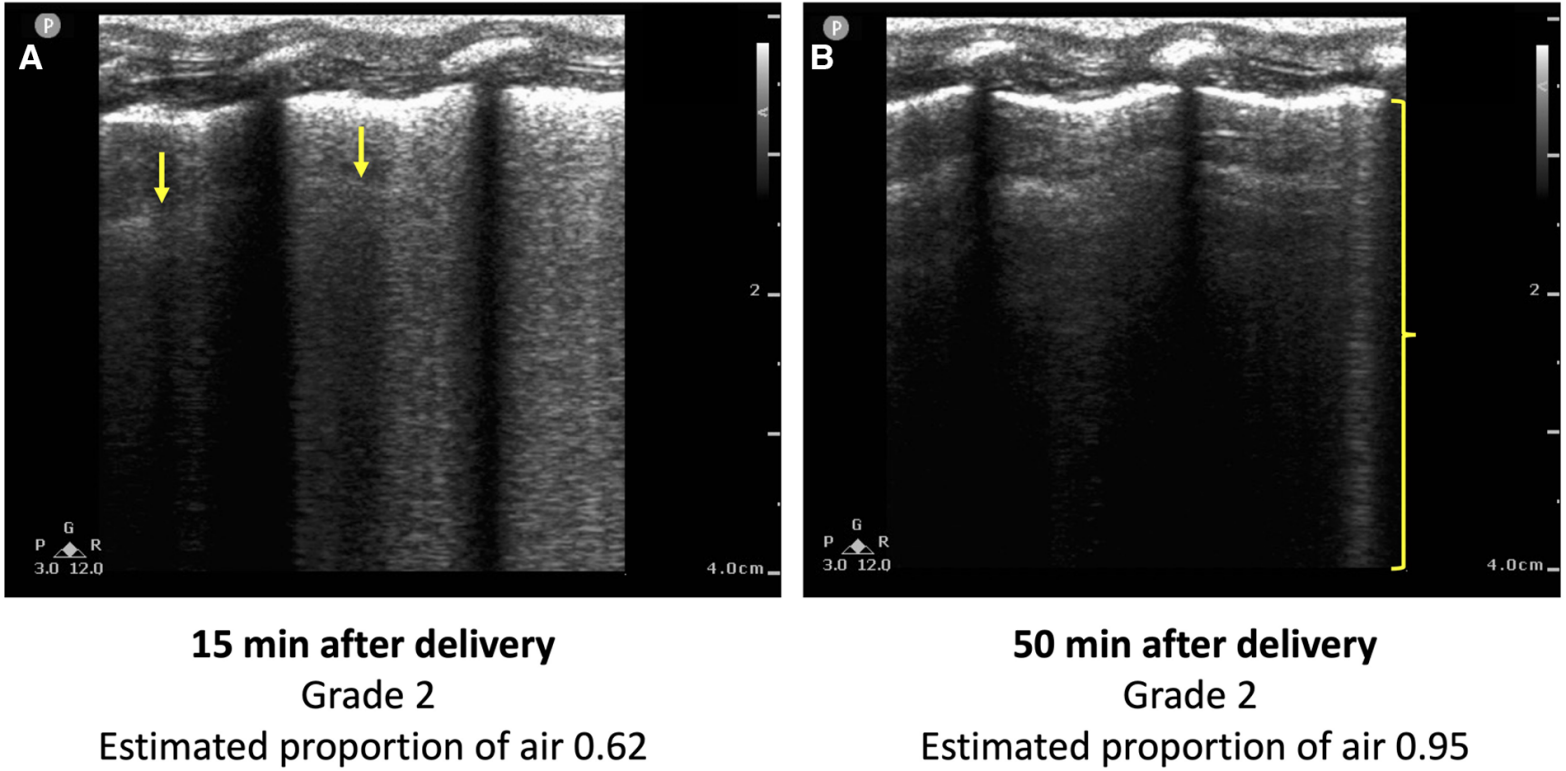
Two lung ultrasound images acquired from the same lamb (A) 15 min and (B) 50 min after the onset of breathing. The image is predominated by coalescence of B lines, which are associated with retained lung liquid and RDS. One B line is visible in (B) (bracket), but the image is predominated by A lines. Using conventional LUS grading systems, both images would be classified as a type 2, despite (B) indicating a higher degree of lung aeration than (A). The estimated proportion of air, calculated using a previously developed technique from the coefficient of variation of pixel intensity, is greater in (B) (0.95 vs. 0.62).
